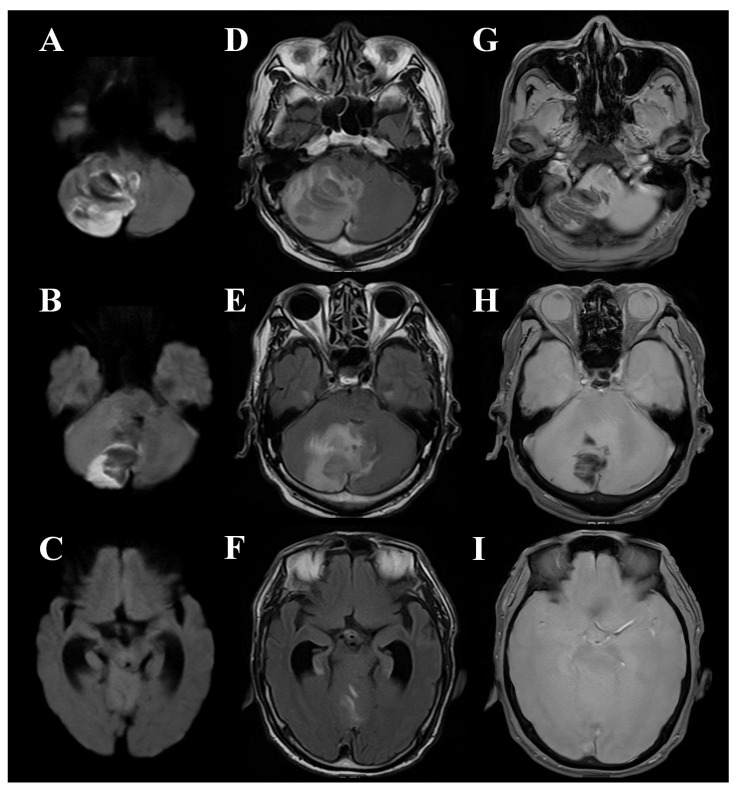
Magnetic resonance imaging findings on admission. (A–C) Diffusion-weighted imaging (DWI) demonstrates an acute right cerebellar infarction with a high signal intensity lesion in the posterior inferior cerebellar hemisphere, associated with mass effect compressing the fourth ventricle. (D–F) Fluid-attenuated inversion recovery (FLAIR) images show hyperintense signals in the infarcted area with surrounding edema and mild hemorrhagic transformation. (G–I) T2*-weighted images reveal patchy low-signal areas within the infarcted region, indicating hemorrhagic components.
